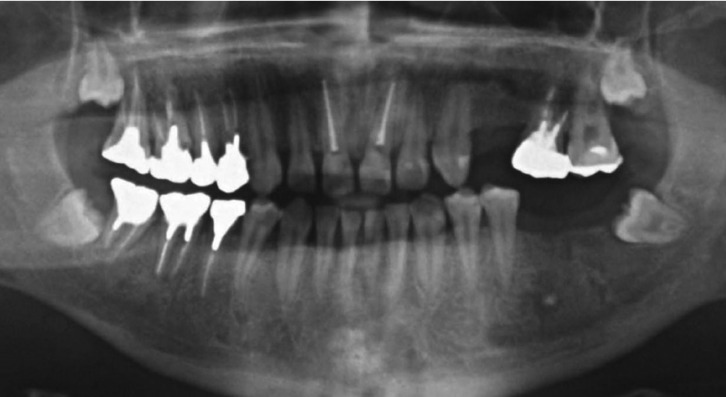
Panoramic radiograph 7 months after surgery.
